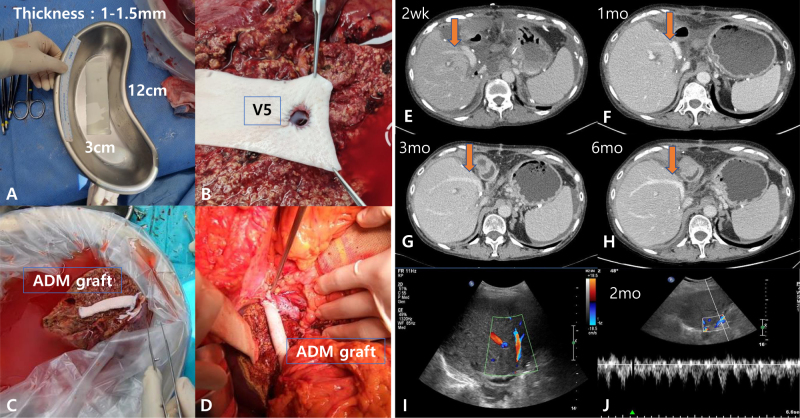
Surgical technique and postoperative imaging of ADM grafts in living donor liver transplantation. (A–D) Surgical technique for ADM conduit preparation. (A) Step 1: A sterile ADM sheet is prepared on the back table. (B) Step 2: The sheet is tailored to the measured dimensions. (C) Step 3: The sheet is fashioned into a tubular conduit using continuous sutures. (D) Step 4: The completed ADM graft is implanted for middle hepatic vein reconstruction. (E–H) Serial CT scans at 2 weeks, 1, 3, and 6 months show persistent graft patency (orange arrows). (I, J) Doppler ultrasonography at 2 months demonstrates a triphasic waveform and stable flow.
